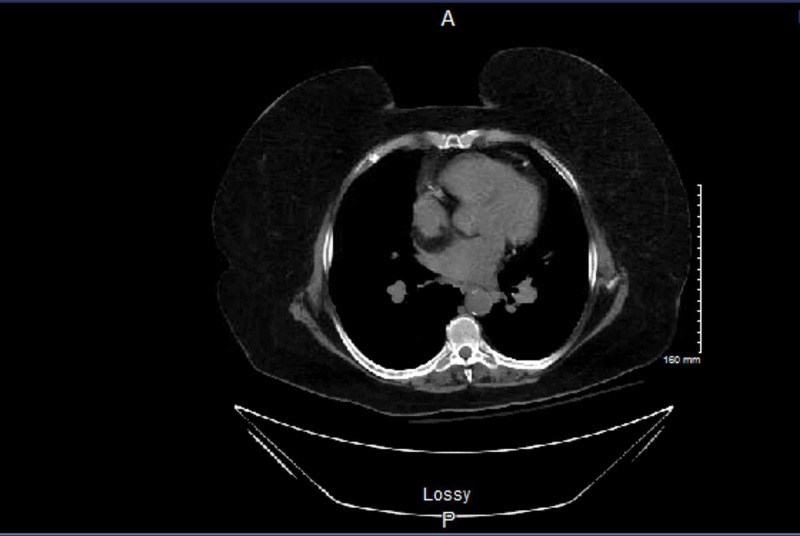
CT abdomen showing an 18 mm cyst upper pole right kidney with a parapelvic cyst measuring 18 mm x 18 mm.
